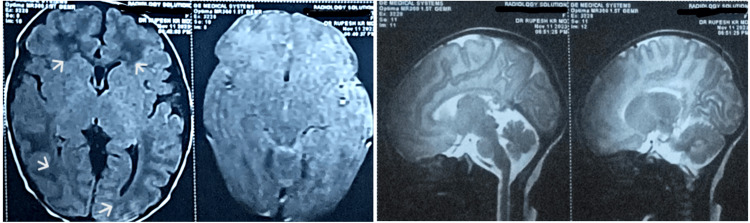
Brain MRI showing mild hypoxic changes in bilateral fronto-parietal and occipito-parietal regions White arrows show the hypodense areas MRI: magnetic resonance imaging
